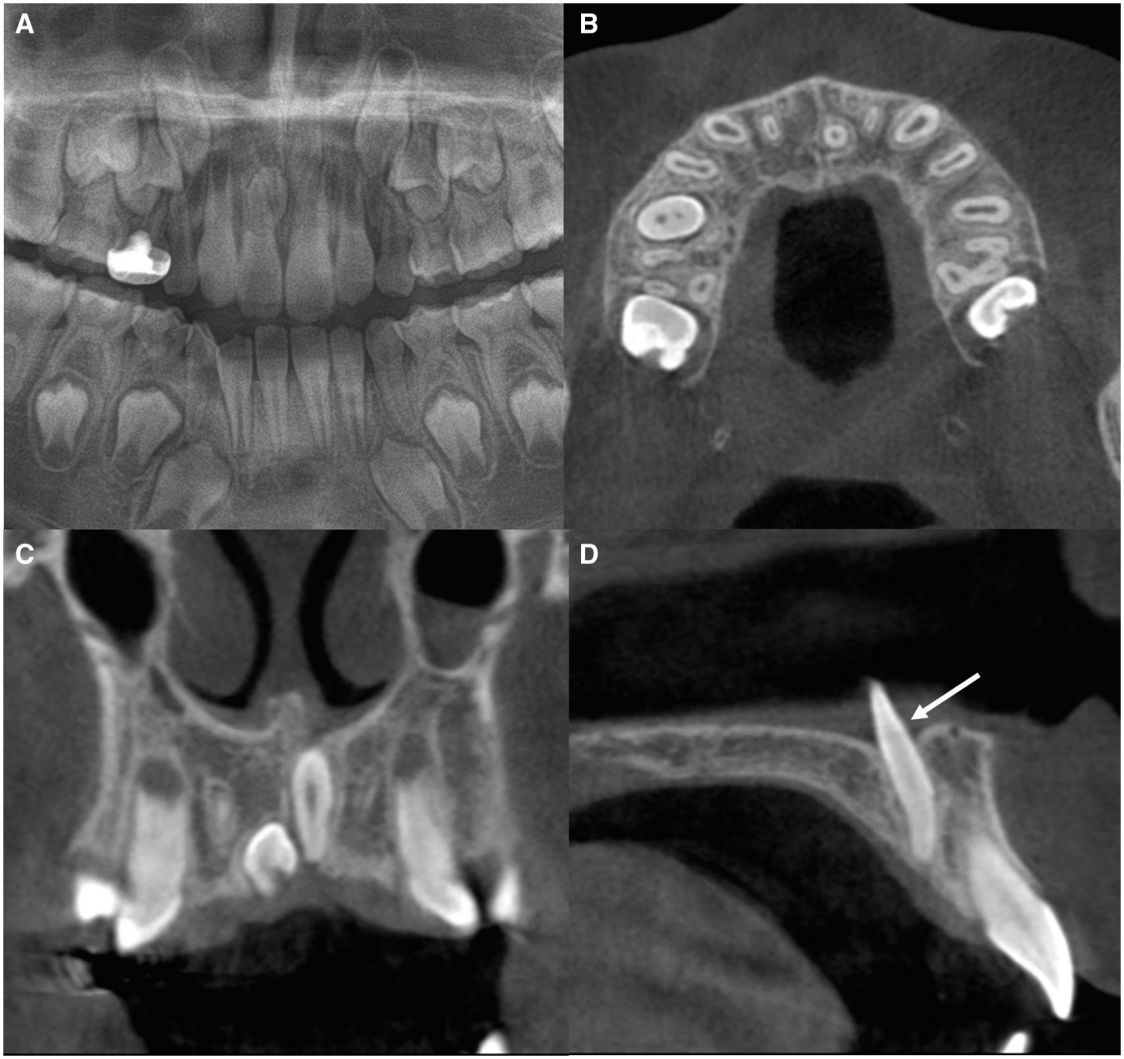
Example of elongated mesiodens in case 13. (A) Cropped panoramic radiograph. (B-D) Cropped CBCT images (axial, coronal, and sagittal views, respectively). Note the elongation in the crown portion of mesiodens within the nasopalatine canal and nasal cavity floor (D, arrow).
